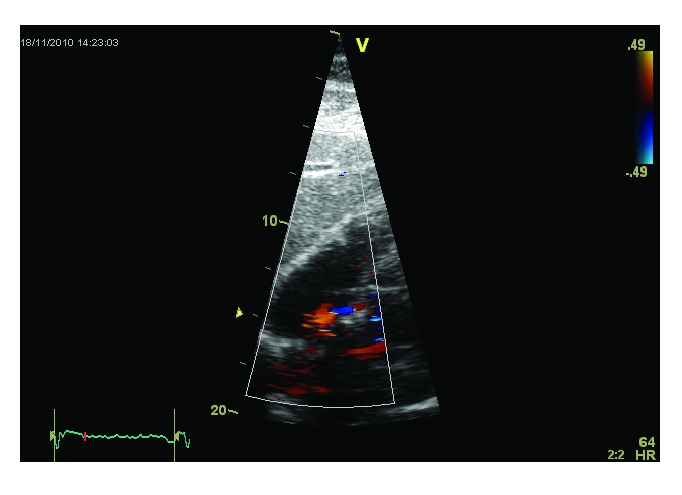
Transthoracic echocardiography, subcostal view: a small interatrial left to right shunt was suggested by the presence of a turbulent flow in color Doppler examination at the level of the interatrial septal aneurysm
